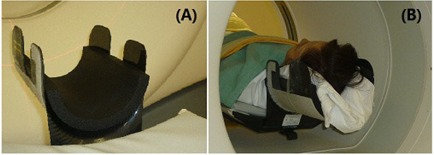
A head immobilization device (U‐shape) used in diagnostic PET/CT imaging: head holder (a) and 1 inch thick foam padding materials; patient setup (b) in the head holder during PET/CT imaging.
